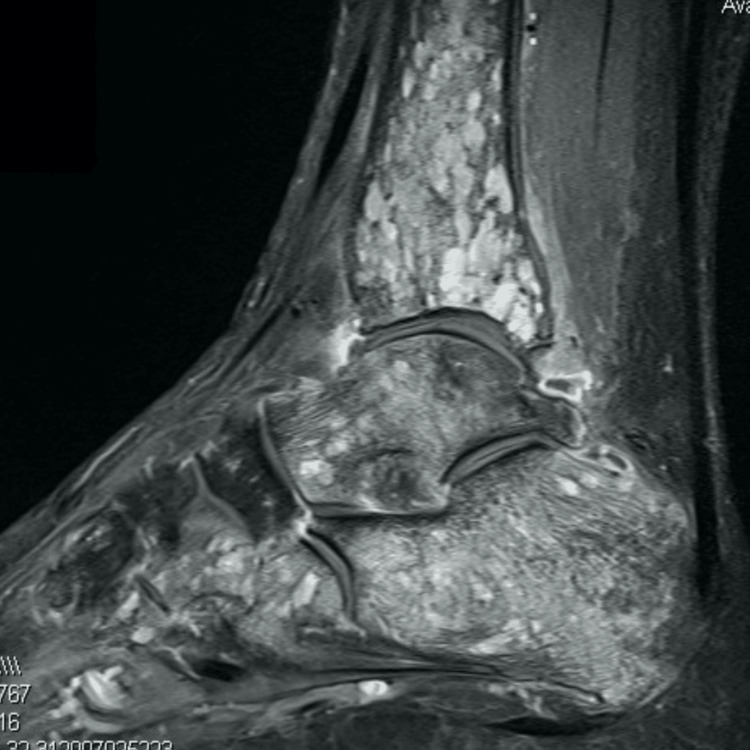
Sagittal contrast-enhanced MRI (gadolinium) showed multiple additional lesions in the hindfoot and tarsal bones, in addition to the previously identified lesions.
